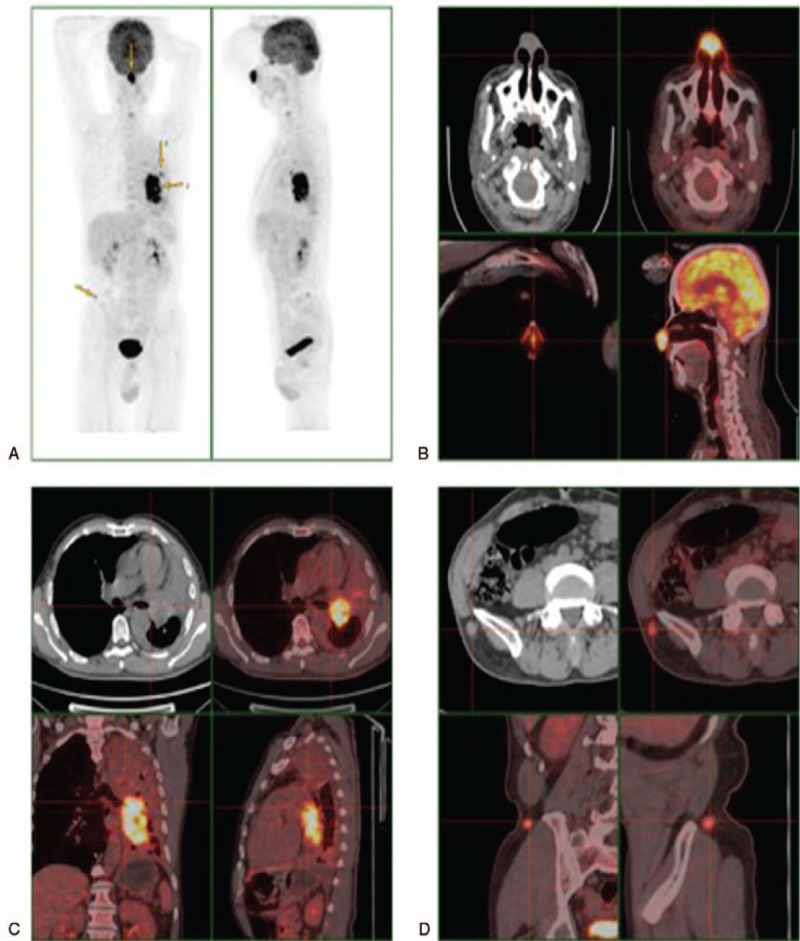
PET-CT scan of the patient, which revealed the nasal tip and suspected buttock metastasis of primary lung cancer. A, whole body scan; B–D, skull, lung, and buttocks scan, respectively, hypermetabolic activities were seen in these areas.
